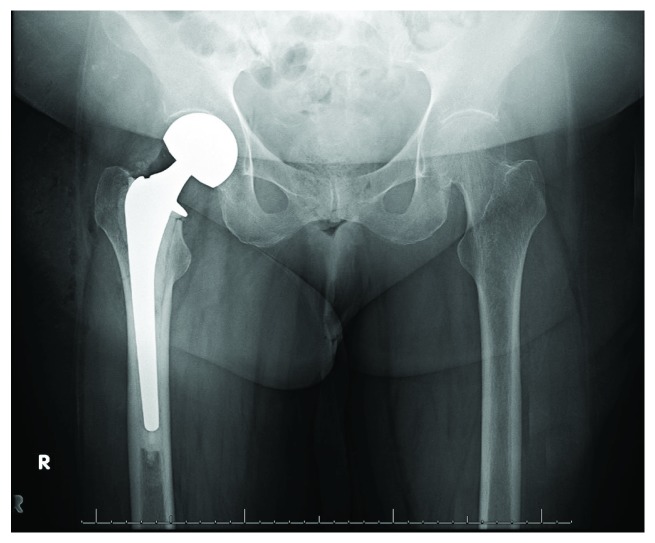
AP pelvis radiograph immediately postop following right hip bipolar hemiarthroplasty.
